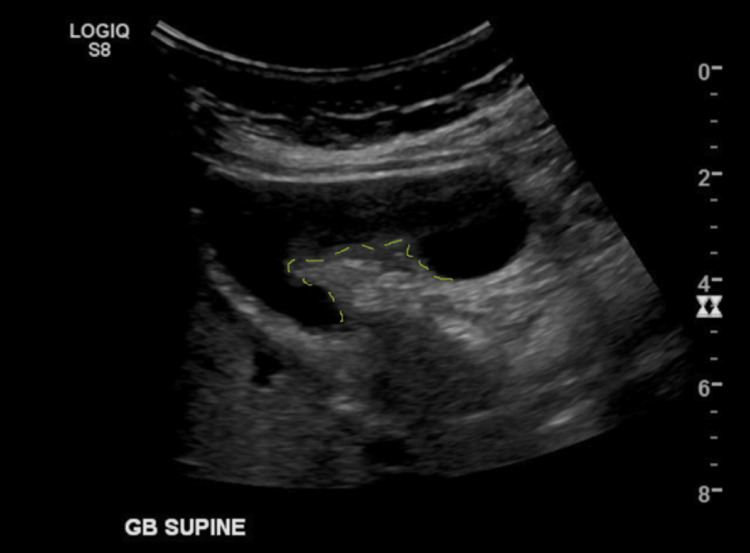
Unusual appearance of the gallbladder on ultrasound which was reported as possible adenomyomatosis
